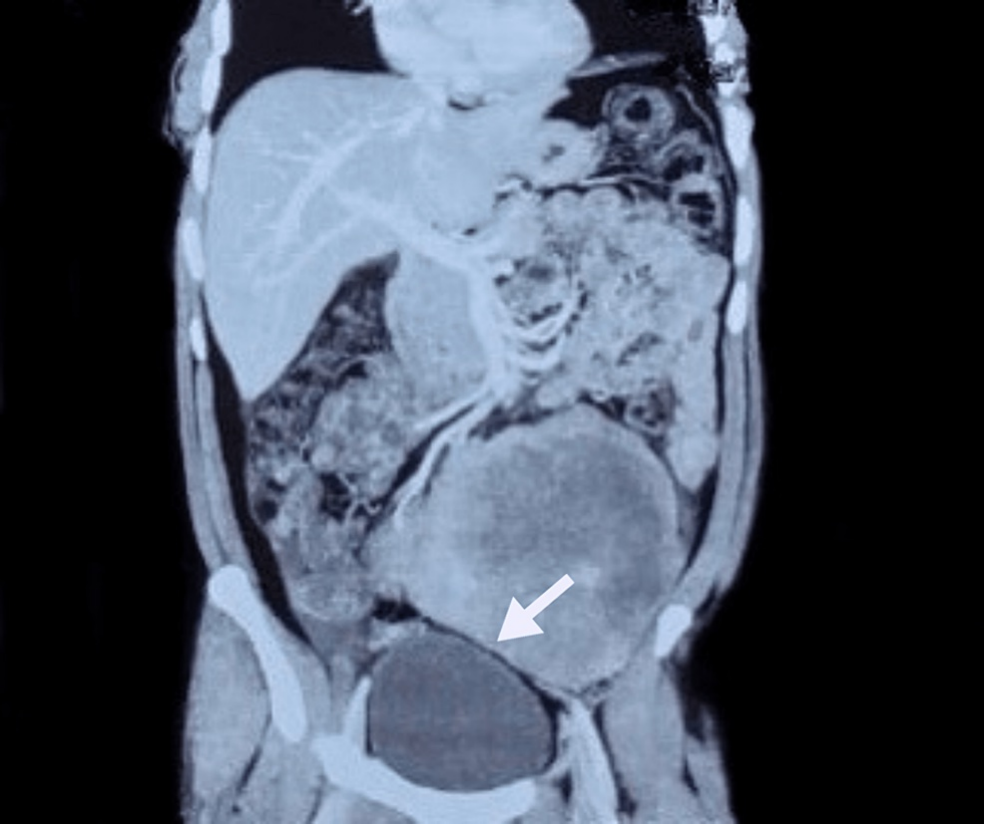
Coronal section of CT scan shows tumor mass compressing the dome of the urinary bladder.
